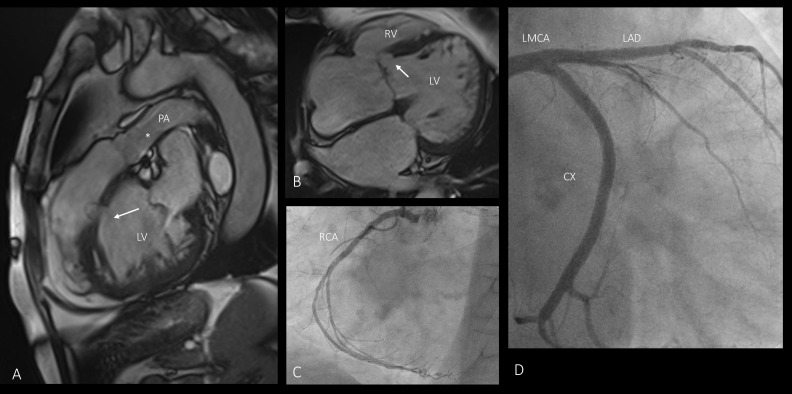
A 77-year-old male with a diagnosis of Double Inlet Left Ventricle (DILV) and Chronic Coronary Syndrome (CCS). He has a history of smoking, diabetes, stage II chronic kidney disease and hypothyroidism. He suffers from chronic atrial fibrillation, for which he has undergone numerous electrical cardioversions (ECVs) and is on AVK treatment. At the age of 57, he underwent coronary angioplasty and stent implantation on the left anterior descending artery (LAD). (A) MRI shows a large ventricular septal defect (➞) and hypoplasia of the pulmonary artery (*), and (B) a rudimentary right ventricle. (C) Angiography shows an irregular right coronary artery without critical stenosis. (D) The left main coronary artery is free from stenosis; the left anterior descending artery presents a good result from previous stenting in the proximal section; and the circumflex branch, codominant, is free from stenosis. CX—circumflex branch; LAD—left anterior descending artery; LMCA—left main coronary artery; LV—left ventricle; PA—pulmonary artery; RCA—right coronary artery; RV—right ventricle.
